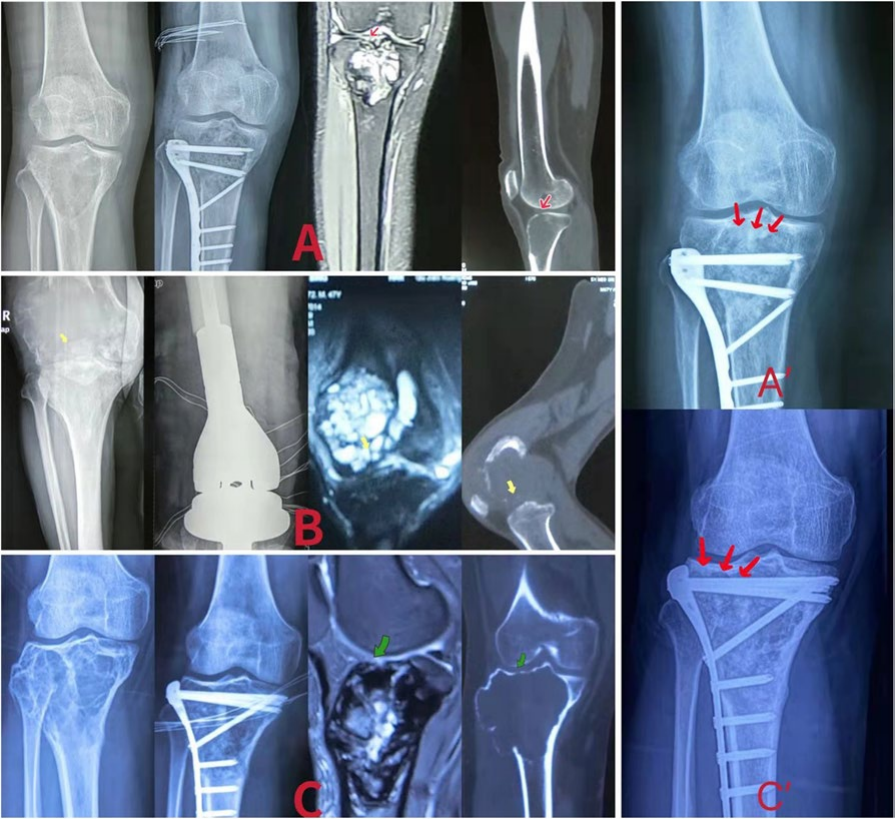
A On MRI and CT, we could see that the destruction of the articular surface of patient A was much less than 50% of the total articular surface (red arrow). The destruction of the articular surface was not obvious. In this case, we believed that the postoperative joint function would not be greatly affected. So we chose the surgical procedure, ‘triple clear’ (TC). At 3 years after surgery, we could see that the patient’s joint morphology was basically the same as that before surgery, and no obvious postoperative arthritis was observed. In the areas where there was the destruction of the articular surface before surgery, obvious autogenous bone repair response could be seen (A’). B All the articular surface of the femur lateral condyle in the patient B was destroyed. The overall range of the damage exceeded 50% of the total articular surface (yellow arrow). At this point, we believed that segmental resection with prosthesis reconstruction was the only option. C We could see that patient C had a fairly large lesion, which even broke through the interosseous compartment. It was graded as Companacci III. However, the destruction of the articular surface was not more than 50% of the overall articular surface (green arrow). In this case, we also chose the TC. At 2 years after surgery, we could see that the patient’s joint morphology was consistent with that before surgery, and no obvious manifestations of arthritis or joint collapse were observed. The subchondral bone repaired obviously. The bone density of the residual cavity also increased significantly (C’)
